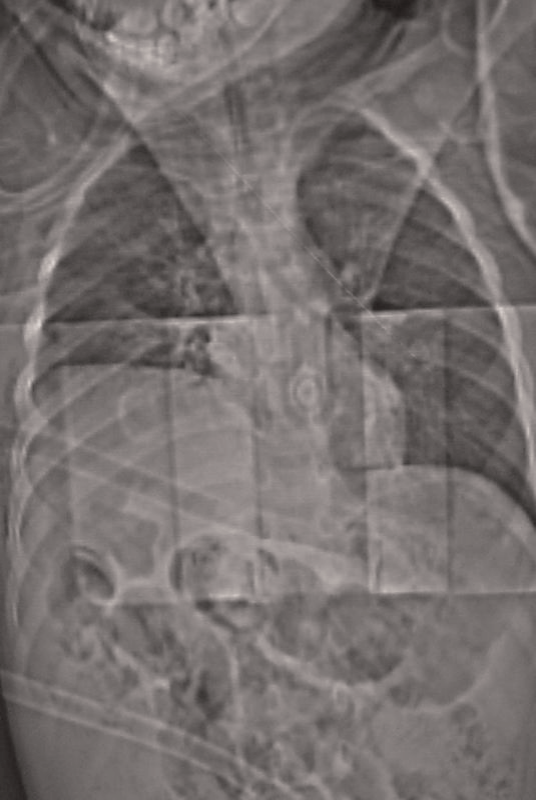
X-ray chest showing elevated right dome of diaphragm.
